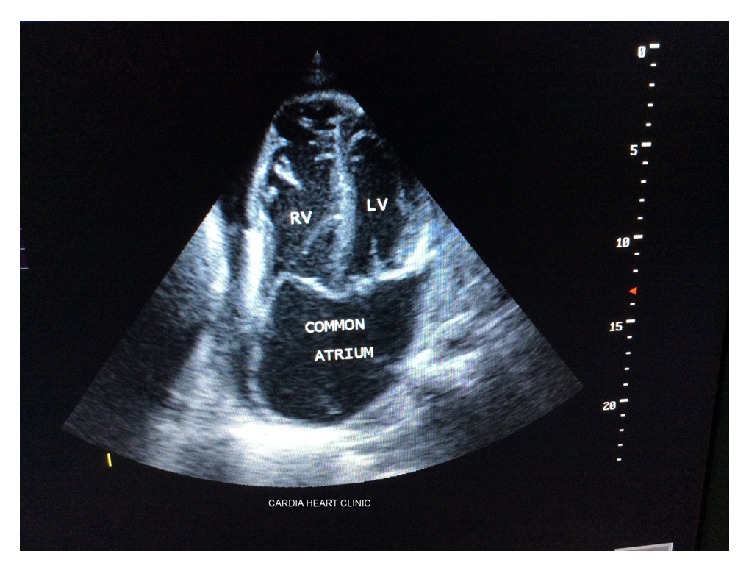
Two-dimensional echocardiogram apical 4-chamber view of the common atrium.
